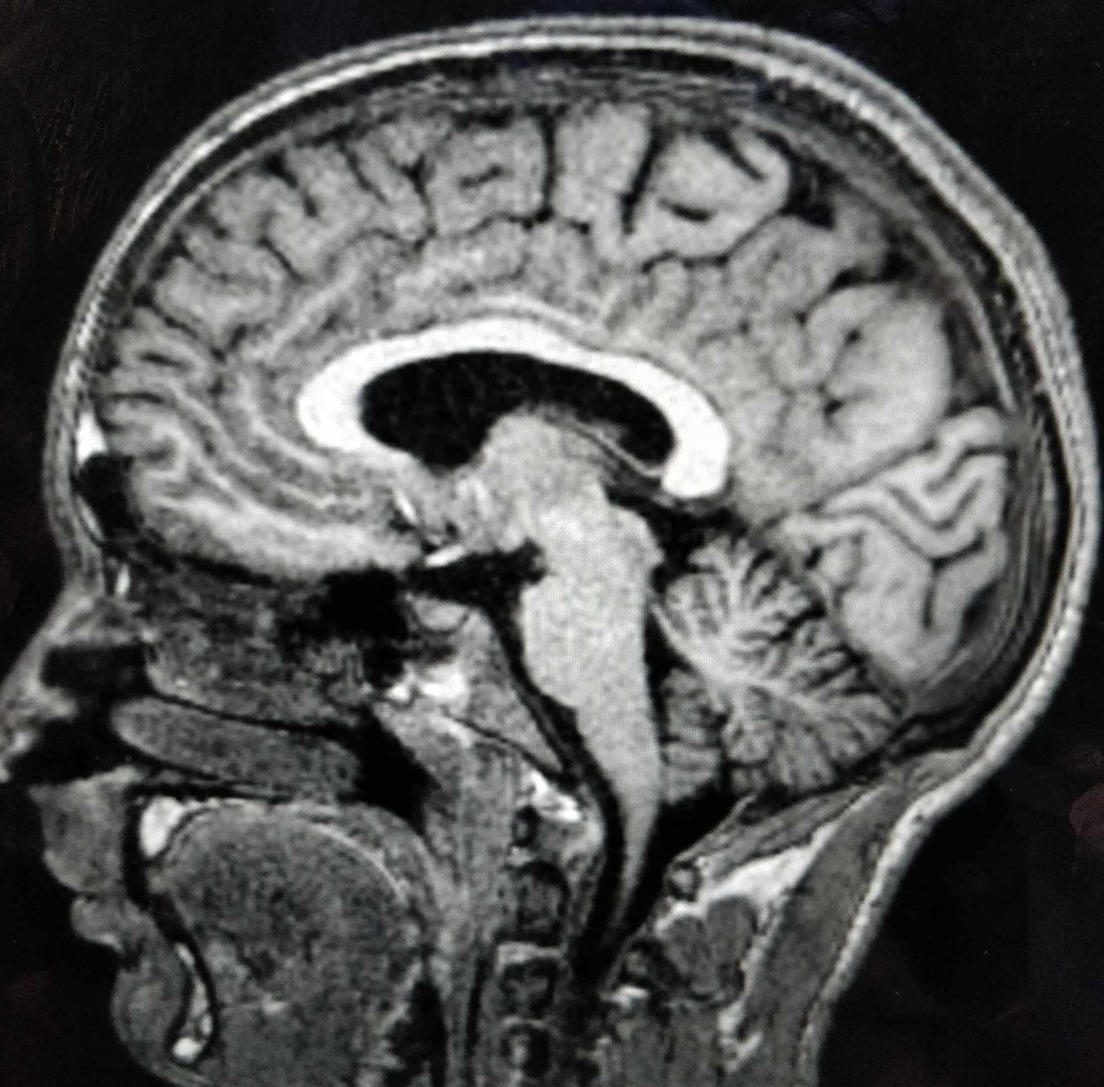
Saggital section of the T1W sequence of MRI brain T1W, T1-weighted; MRI, magnetic resonance imaging No structural abnormality is seen in the brainstem.
